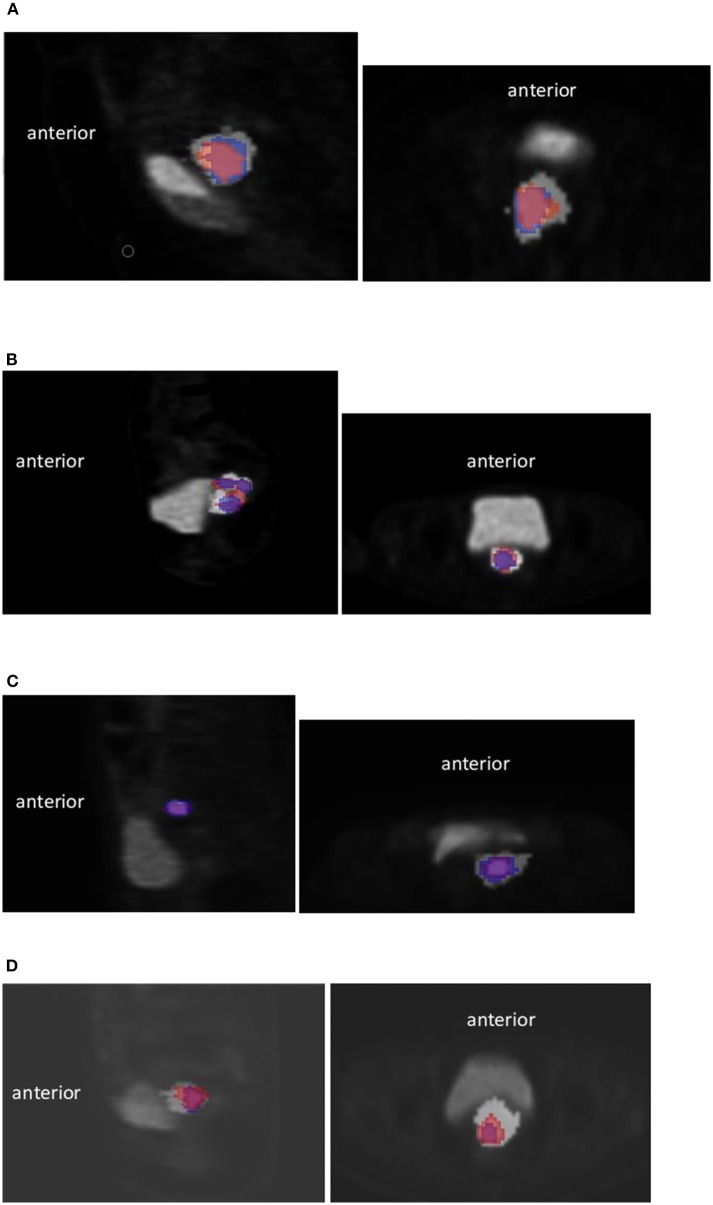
Examples of registration using the 3D Slicer TM Expert Automated Registration module in 4 different patients. Initial high 18F-FDG uptake sub-volume (V1) in blue and relapse V2 in red. Sagittal view (left) and axial (right) (A) for this particular case, a manual correction of the registration due to a different bladder filling was necessary (Dice = 0.73, OF = 0.77, X = 0.70, Y = 0.77), (B) Case with V2 larger than V1 (Dice = 0.62, OF = 0.80, X = 0.80, Y = 0.50), (C) Case with small V1 and V2 (Dice = 0.72, OF = 0.82, X = 0.64, Y = 0.82), (D) Case with V2 larger than V1 (Dice = 0.76, OF = 0.90, X = 0.90, Y = 0.65).
